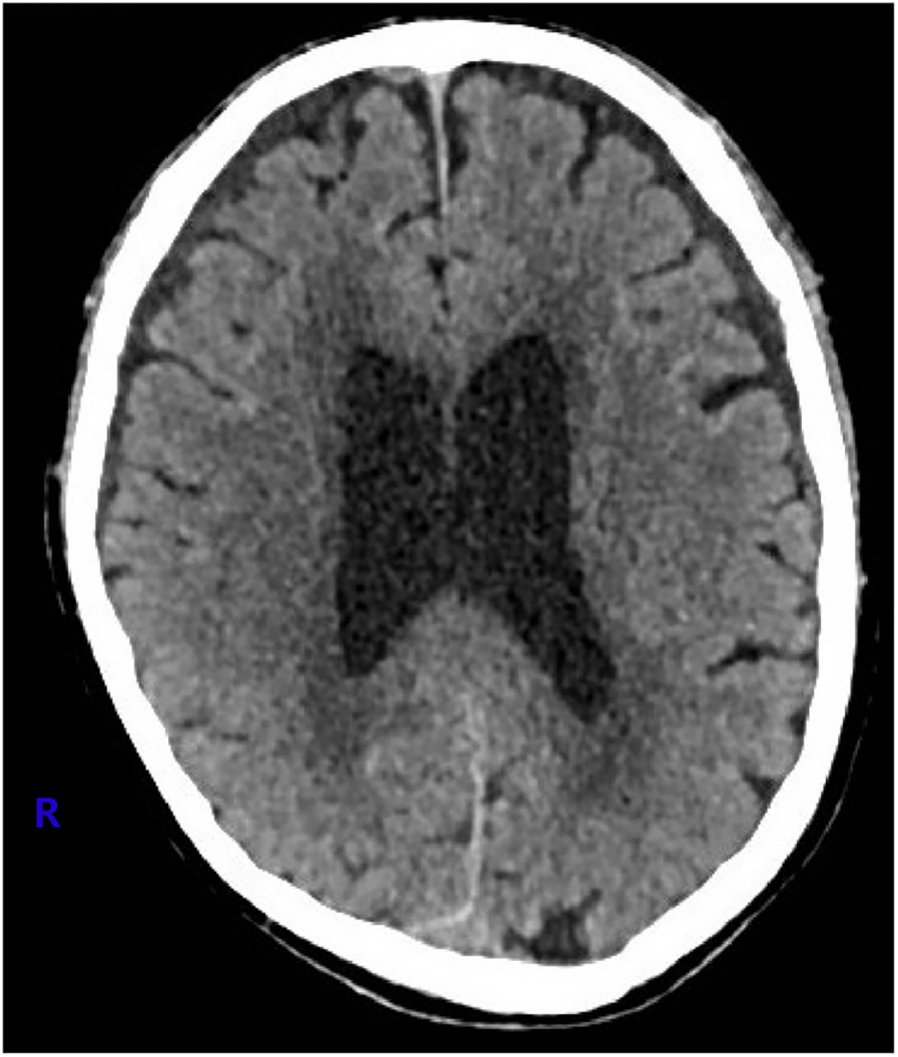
At one year after the procedure, the cranial computed tomography image showed no sign of subdural hematoma.
